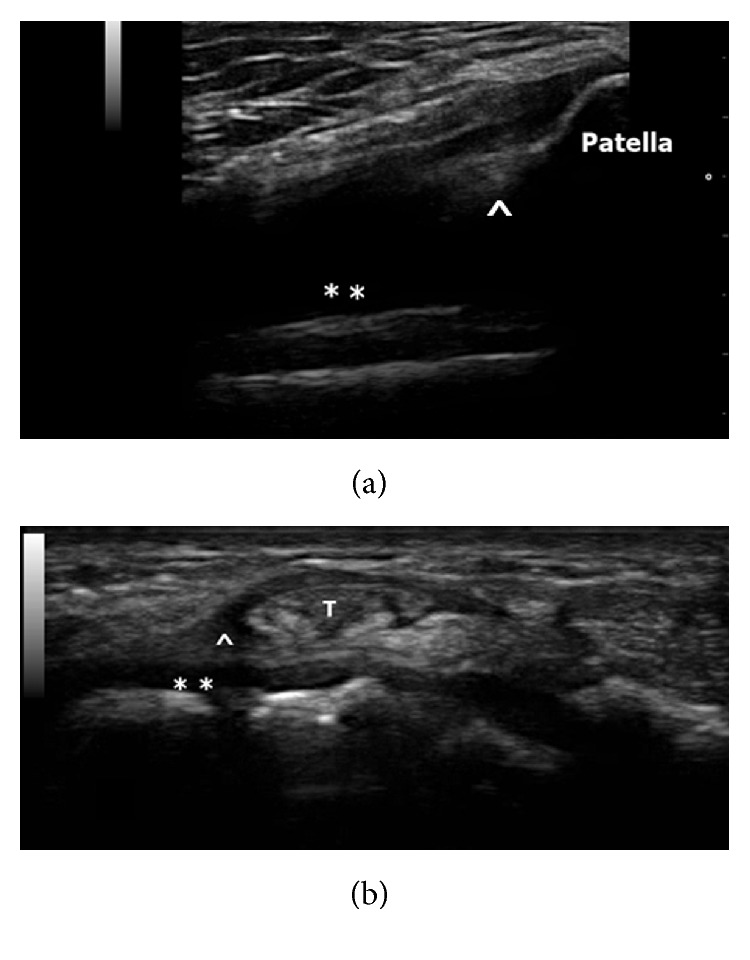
Ultrasound of knee and wrist. (a) Longitudinal scan of knee demonstrated hypoechoic joint fluid (*∗∗*) and synovial hypertrophy (∧) at suprapatellar recess. (b) Transverse scan of dorsum of wrist showed hypoechoic effusion (*∗∗*) and rim of fluid compatible with tenosynovitis (∧) of extensor tendon (T).
